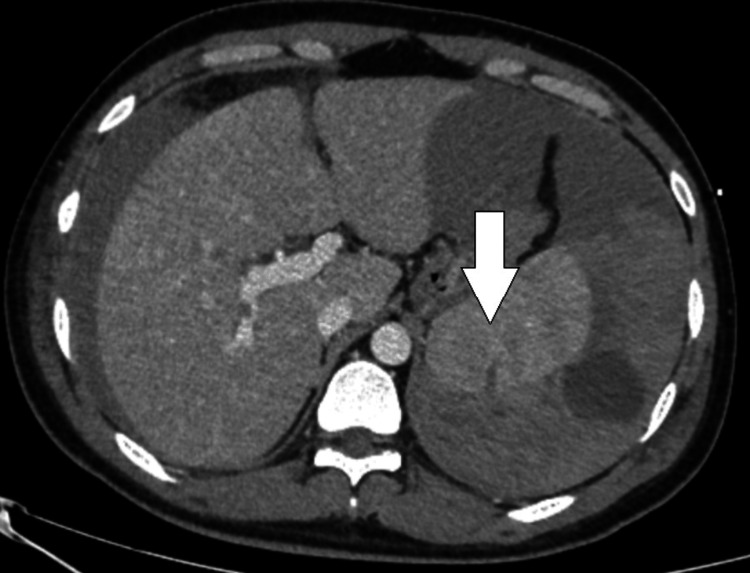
CECT abdomen on day 4, showing a decrease in the size of the hematoma CECT: Contrast-enhanced computed tomography
